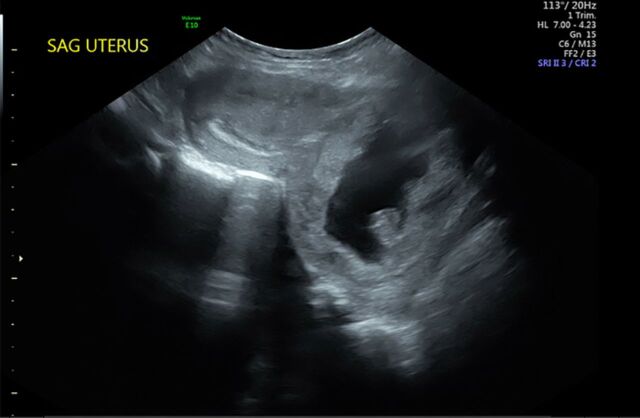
Sagittal (SAG) ultrasound of cervical ectopic pregnancy at 13 weeks 3 days’ gestation, demonstrating an hourglass-shaped uterus with a ballooned endocervical canal, gestational tissue at the level of the cervix, and empty uterine cavity.
